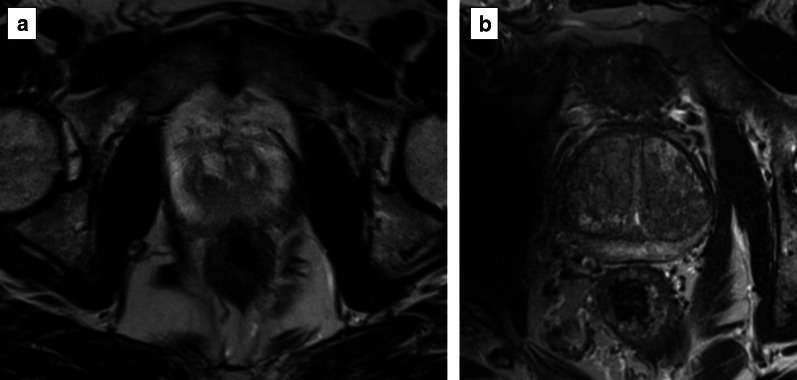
Axial T2-weighted images showing artefacts from movement (a) and metallic implants in the right hip (b)
